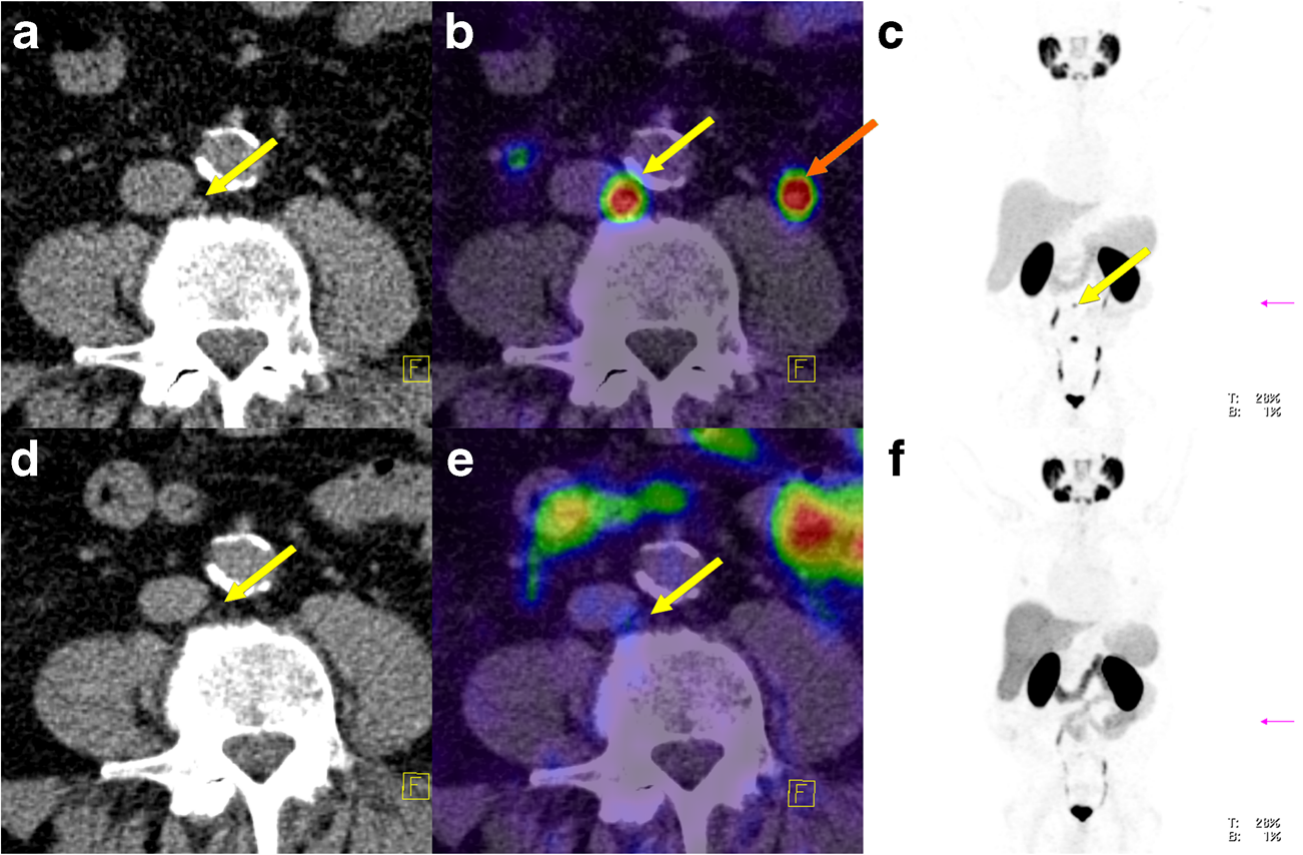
Lymph node metastasis in an example patient (patient 9) without ADT (a–c) and during ongoing ADT with a complete PSA response (d–f) 228 days after the first scan (*orange arrow* left ureter). a Low-dose CT image without ADT. b Fused PET and CT image without ADT. c Maximum intensity projection image of the PET data without ADT. d–f same as a–c but during ongoing ADT
